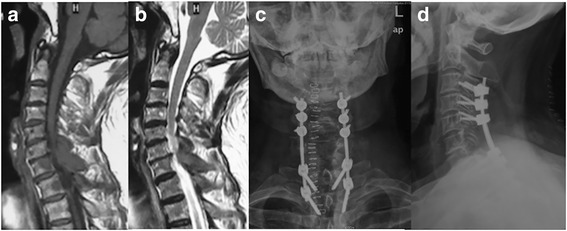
A 66-year-old male with prostate cancer was admitted because of severe neck pain and lower extremity weakness. Mid-sagittal T1 (a) and T2 (b) MR image showing multiple level lesions in the cervicothoracic junction area and posterior encroachment of spinal canal. Anteroposterior (c) and lateral (d) radiographs were obtained after posterior tumor resection, decompression, and fixation. The patient’s pain and neurological deficit were significantly improved
